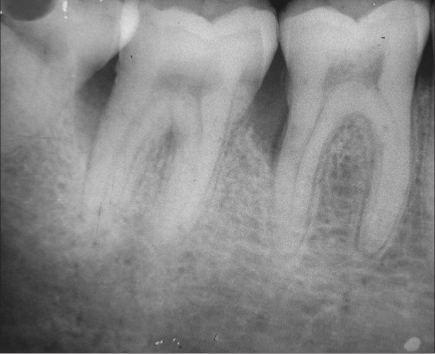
Radiograph showing infrabony defects distal to 46 and 47
